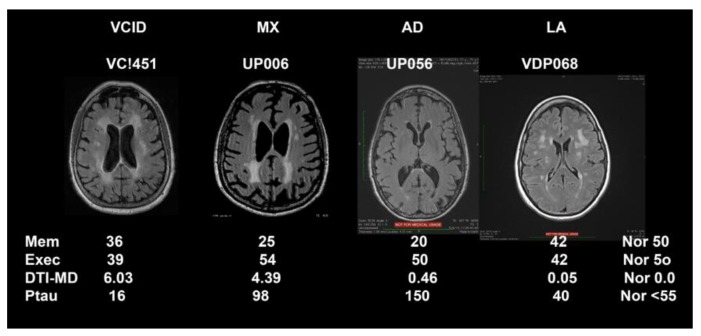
MRI fluid attenuated inversion recovery (FLAIR) sequences on top and biomarker values for each of the patients from the different diagnostic categories. The vascular cognitive impairment and dementia (VCID) patient has a normal memory and executive score. The diffusion tensor imaging-mean diffusion (DTI-MD) is elevated with a normal of 0.0 since these are z-scores, and the Ptau is normal. The mixed dementia (MX) patient shows a low memory and normal executive function, an elevated DTI-MD, and an increased Ptau. The Alzheimer’s disease (AD) patient has very low memory score with a normal executive function, a normal DTI-MD and an extremely elevated Ptau. The leukoaraiosis (LA) patient has white matter changes on FLAIR, but normal scores for all the biomarkers, including MD. Normal (Nor) values are shown in the right column.
